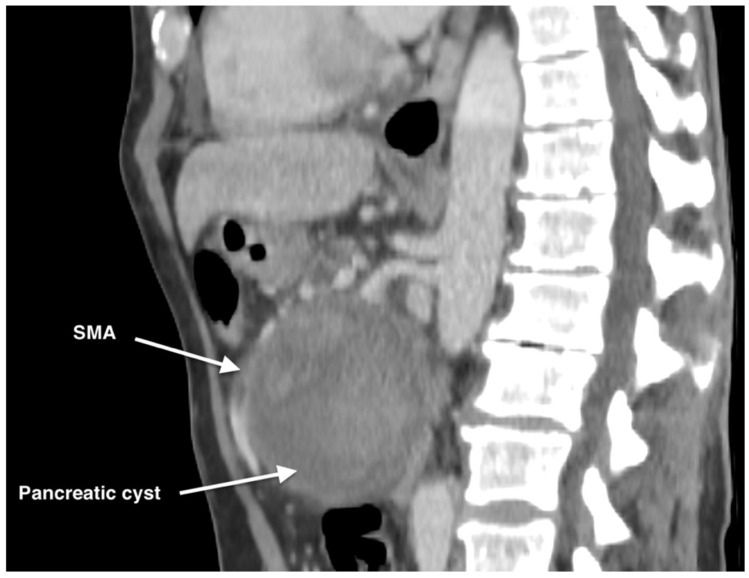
Preoperative CTA aspect of the ruptured SMAA and the pancreatic cyst-sagittal view describing the course of the SMA along the pancreatic cyst.
